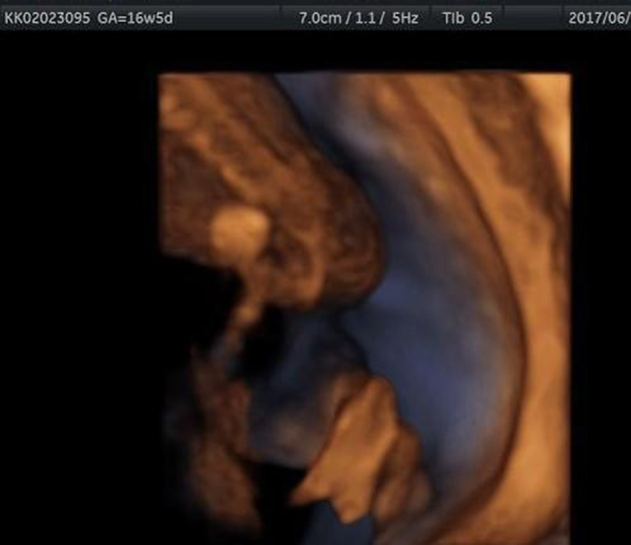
EEC syndrome. Three-dimensional ultrasound in rendering mode demonstrates the lobster-claw foot.
